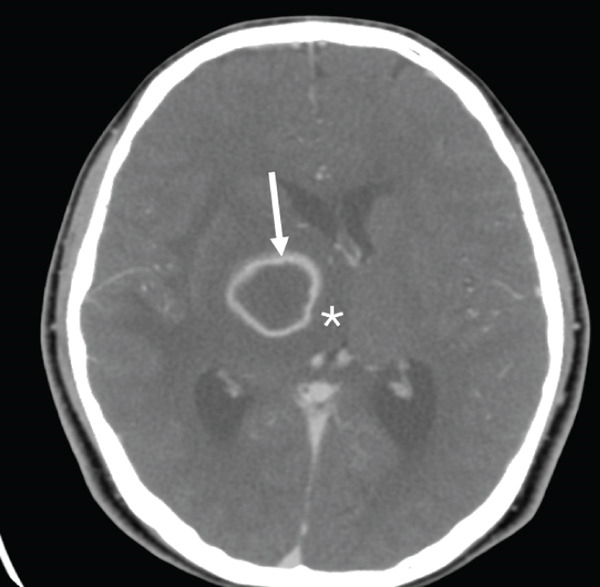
The brain CT image showed a lesion with central necrotic‐colliquative area, surrounded by an external capsule (arrow) and edema (asterisk).
